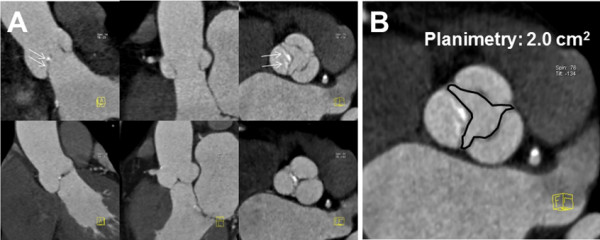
Example of PTAS CTA in aortic valve evaluation. A: Reconstructions at 250 msec (top row) and 400 msec (bottom row) show the aortic valve in open and closed positions. There is mild restriction of the non-coronary cusp opening (arrows). B: Planimetry shows mild aortic stenosis. No motion artifacts were seen in the coronary arteries (not shown).
